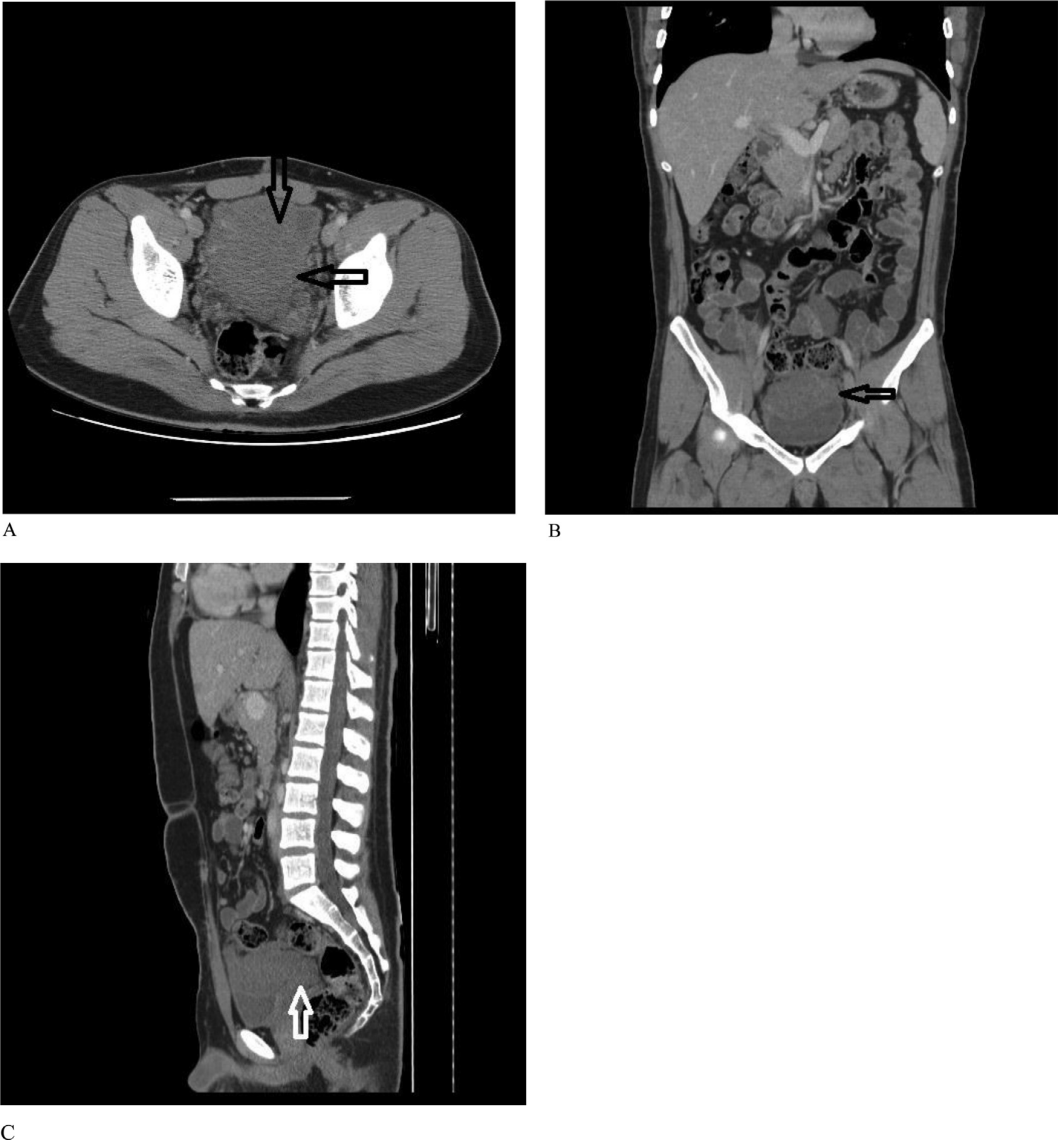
Axial (A), coronal (B), and sagittal (C) abdominopelvic CT scan with IV contrast: a large enhancing heterogeneous mass in the pelvic region between the urinary bladder and rectum (black arrows) with pressure effect and suspicious invasion to posterior and superior walls of the bladder (white arrow).
